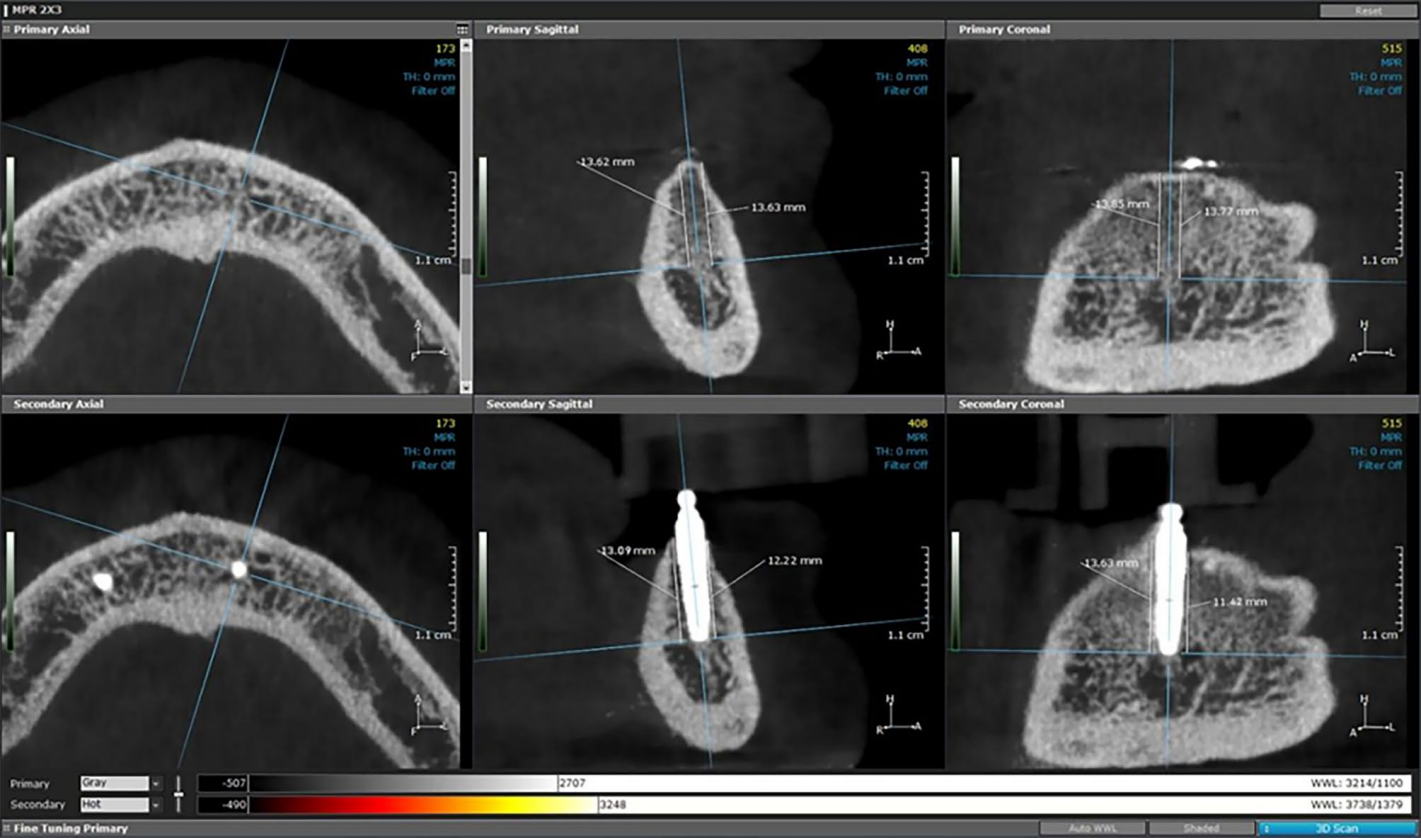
Measurements of peri-implant bone level changes by CBCT
